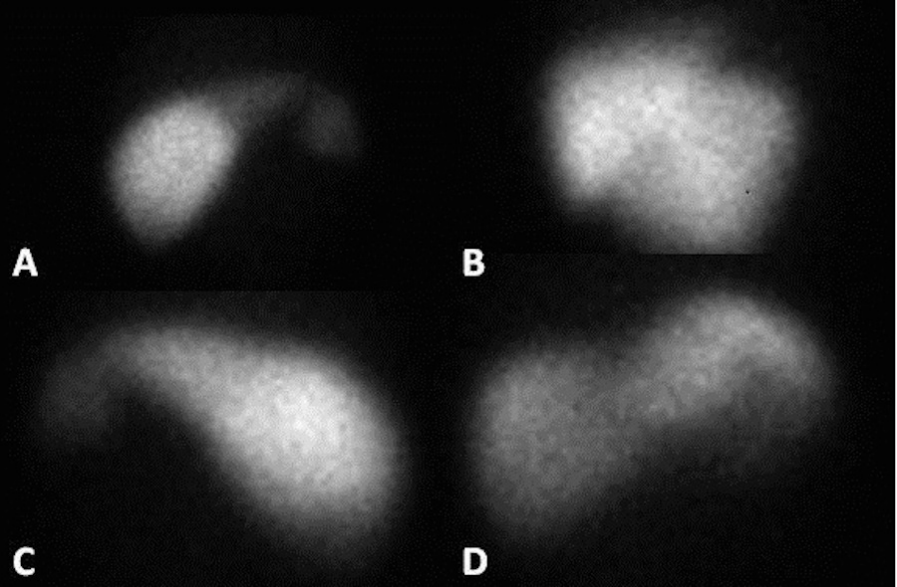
Splenic scintigraphy Splenic scintigraphy using Tc-99m sulfur colloid from a single patient in various projections demonstrating multiple spleens in the right side of the abdomen.
